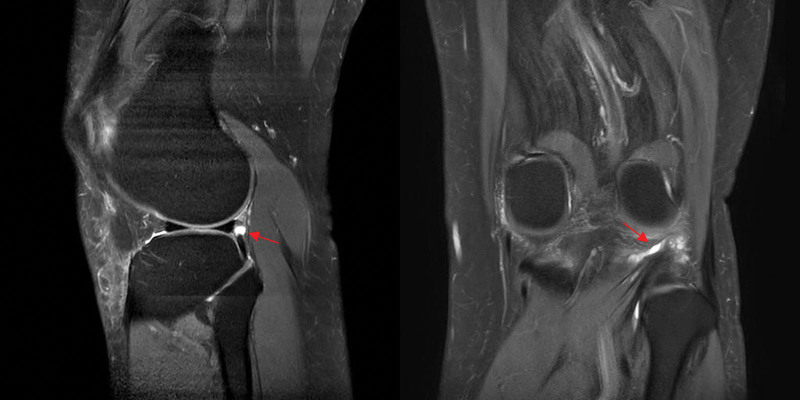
On T2-weighted proton density (A) sagittal and (B) coronal knee magnetic resonance images taken two years after the in-car traffic accident, a partial-thickness tear with intrasubstance ganglion cysts (arrows) is revealed in the musculotendinous junction of the popliteus tendon.
